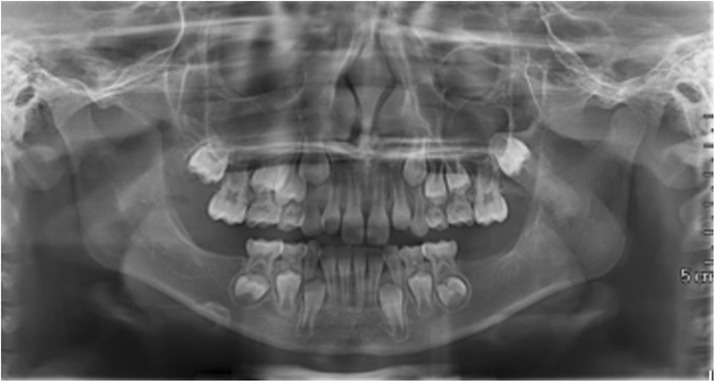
TA, female, diagnosis at age 9. Follow-up at 8 months years *(16-oct-2013)*. Panoramic radiography shows the good aspect of the bone mandibular structures and the absence of relapse
